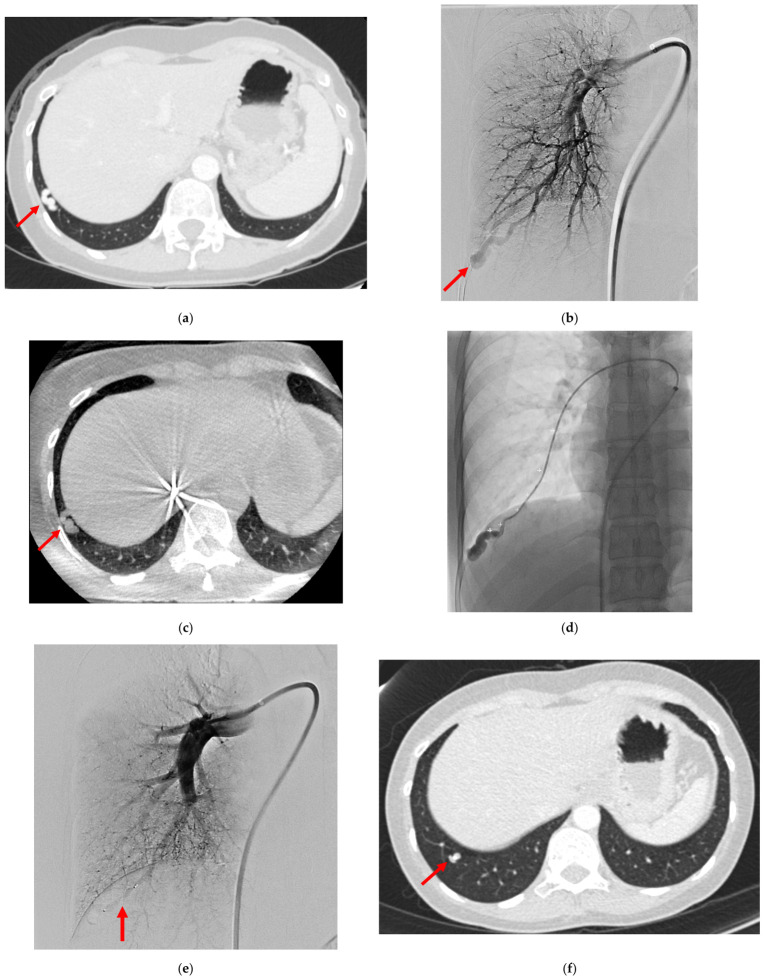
A 37-year-old woman with a pulmonary arteriovenous malformation (PAVM). (a) Preprocedural CT in the lung window shows the nidus of the PAVM (arrow) in the right lower lobe. (b) Digital subtraction angiography (DSA) from the right main pulmonary artery demonstrates the target PAVM (arrow). (c) Noncontrast cone-beam CT (CBCT) depicts the PAVM (arrow) in the same location as on preprocedural CT. (d) Overlay fluoroscopy after CBCT shows the trajectory of the feeding artery from the right main pulmonary artery to the nidus; note how the catheter closely follows the CBCT-derived trajectory. (e) Completion DSA confirms successful embolization with a vascular plug (arrow) and absence of opacification in the draining vein. (f) Follow-up CT obtained at 12 months later demonstrates complete resolution of the PAVM with the vascular plug (arrow) remaining in situ.
